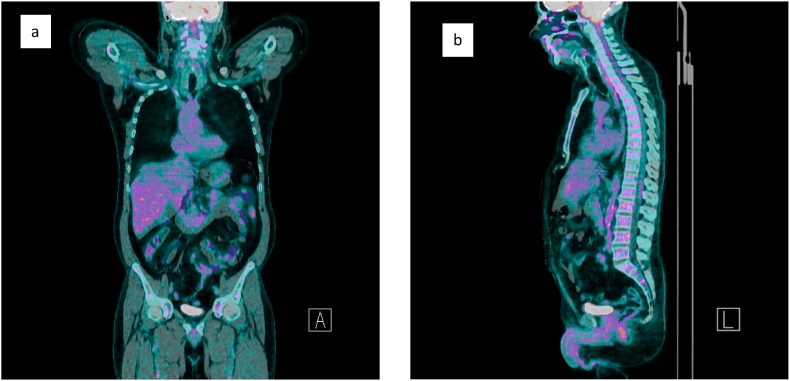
18F- FDG PET after surgey and chemotherapy: there is complete disappearance of the hyperfixations previously reported, demonstrating the complete response to the therapy in progress.
